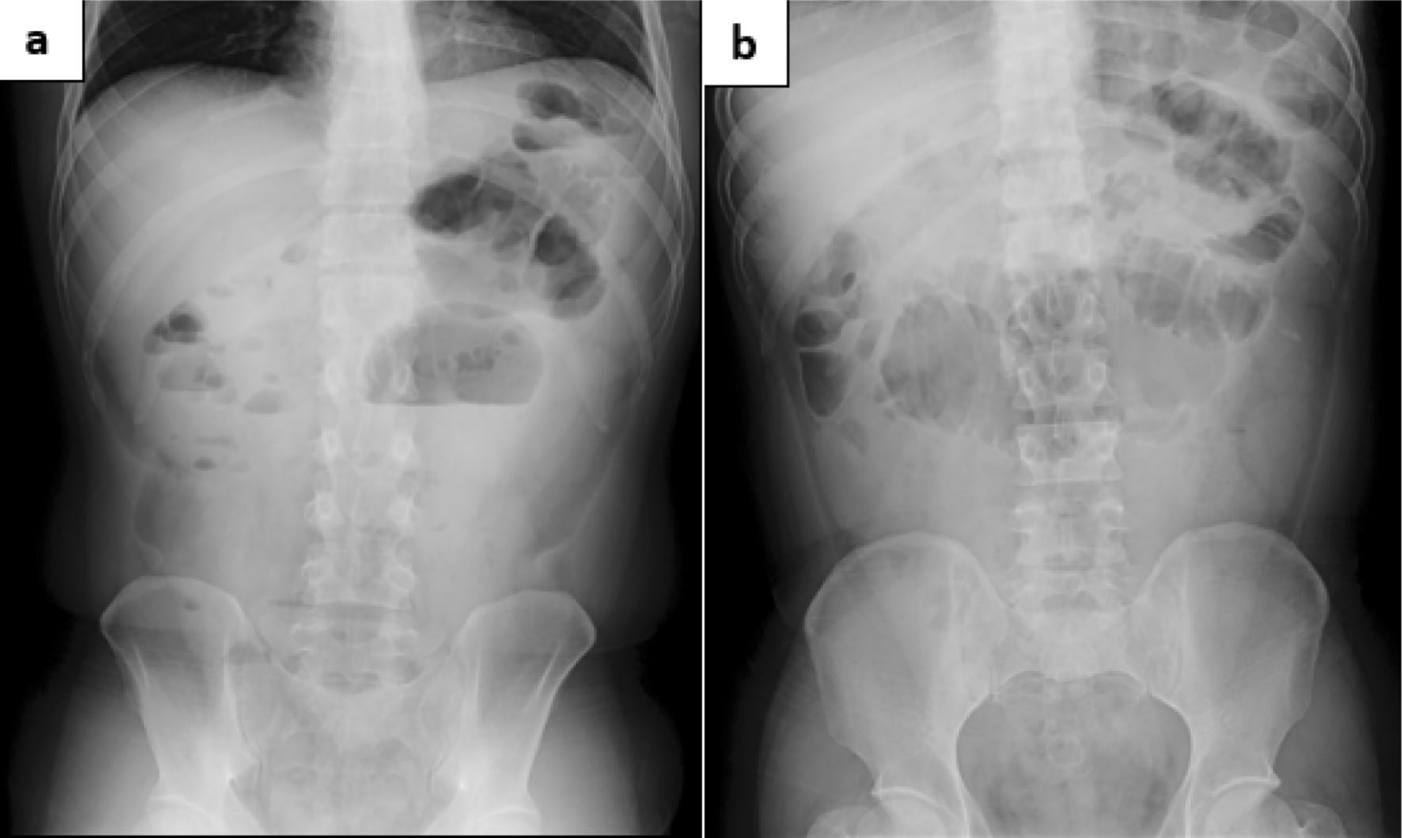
Abdominal radiograph obtained in December 2017. a Standing position. b Decubitus position
